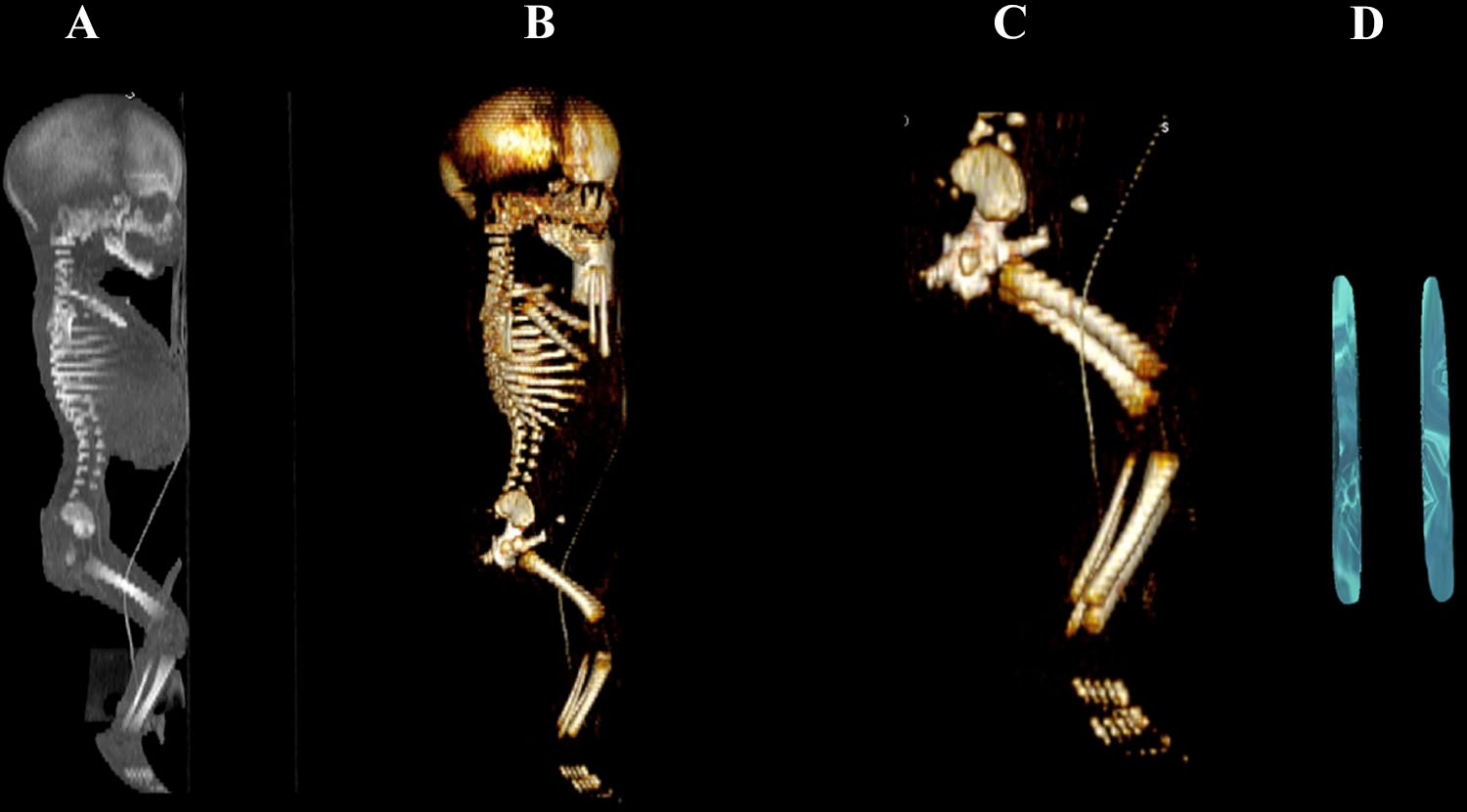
CT of a male fetus aged 19 weeks (in the sagittal projection) recorded in DICOM formats (a), with the sagittal 3D reconstruction (b), with the sagittal projection of the fetal pelvic girdle and lower limbs (c), with primary ossification center of the fibular shaft (d), assessed by Osirix 3.9
